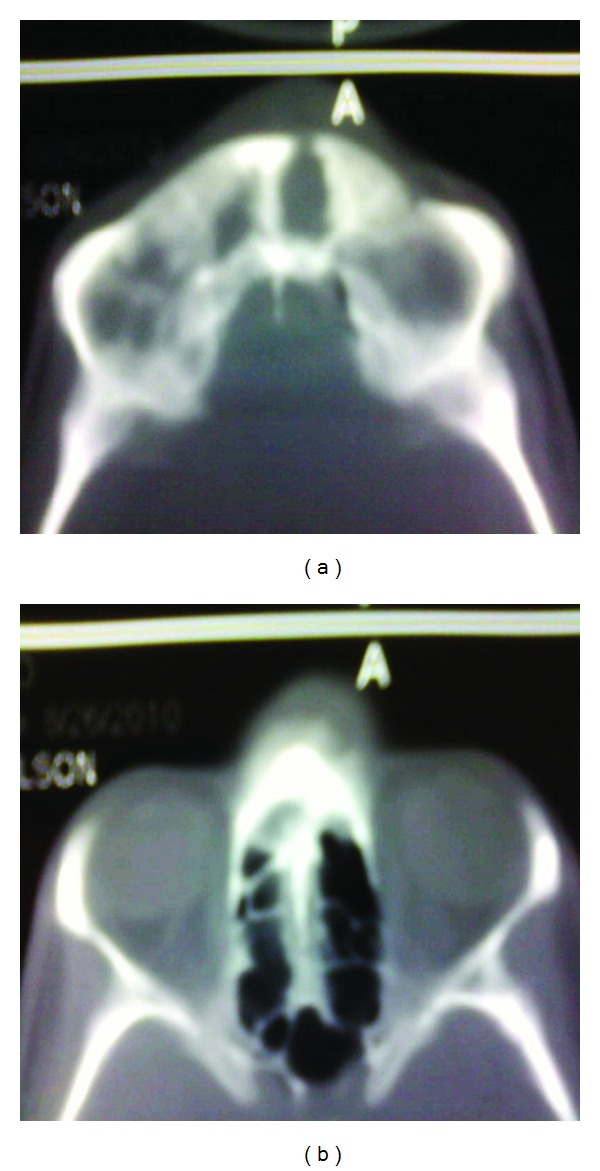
Axial CT scan images of the left frontal sinus tumor with anteroinferior frontal sinus wall erosion. The right frontal sinus contained purulent discharge (noted in the operating room).
